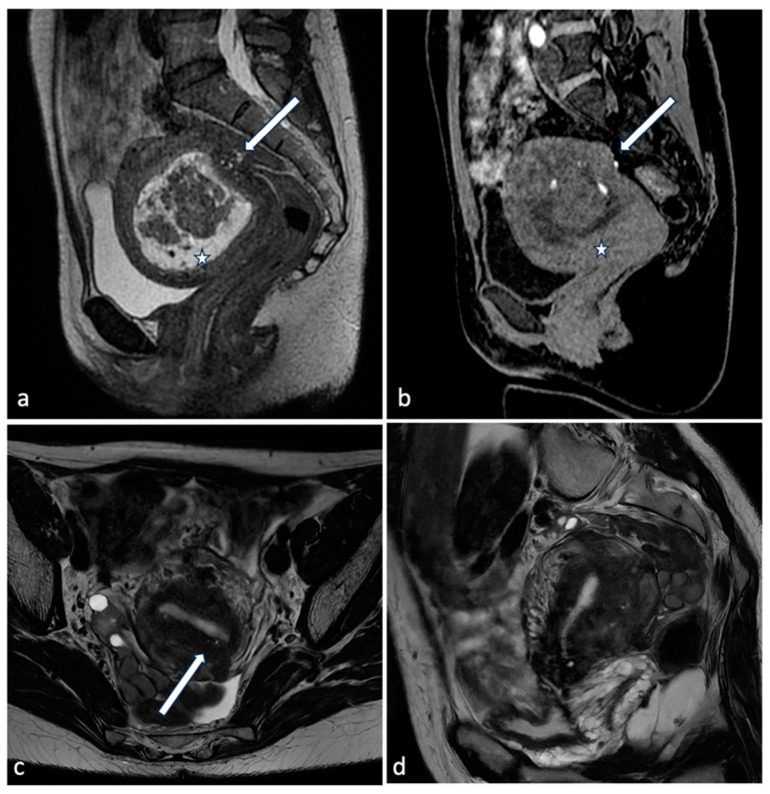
Different endometriosis phenotypes. A 41-year-old patient with a history of infertility, menometrorrhagias and chronic pelvic pain: MRI shows on sagittal CUBE T2WI (a, arrow) an extensive posterior external adenomyosis with bright foci on T1WI (b, arrow). There was associated deep pelvic infiltrating endometriosis and a large submucosal leiomyoma (a,b, star). Another 50-year-old patient with history of metrorrhagias: MRI demonstrates on axial T2WI (c) internal diffuse adenomyosis with hyperintense foci within the JZ (arrow). Sagittal T2 sequence (d) shows a diffuse thickening of the JZ (15 mm), prevalent on the posterior wall.
